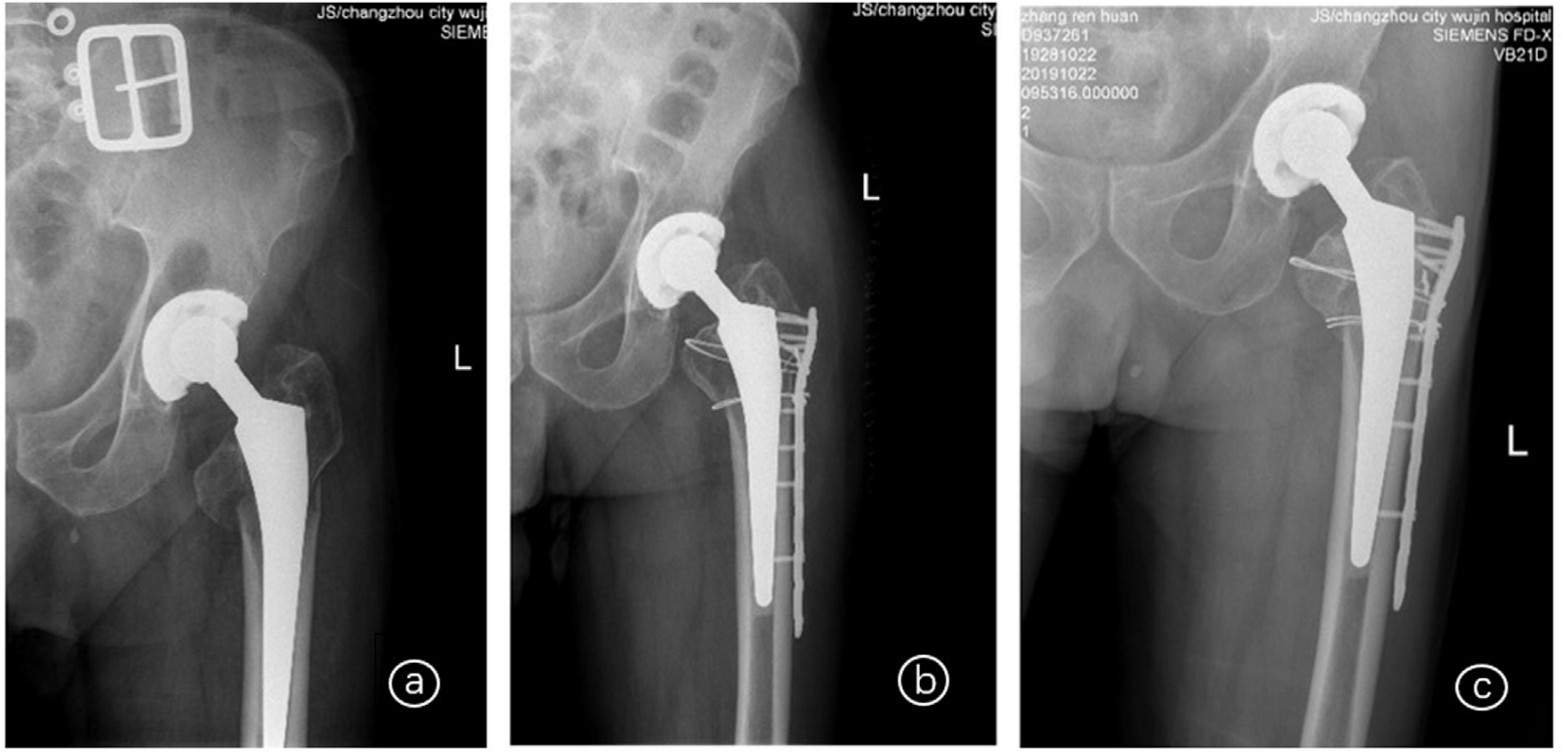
X-rays of an 86-year-old female patient with Vancouver B1 PFF who underwent left hip arthroplasty 14 years and 2 months ago; ⓐ Before surgery; ⓑ 5 days after surgery; ⓒ 1 year and 9 months after surgery.
